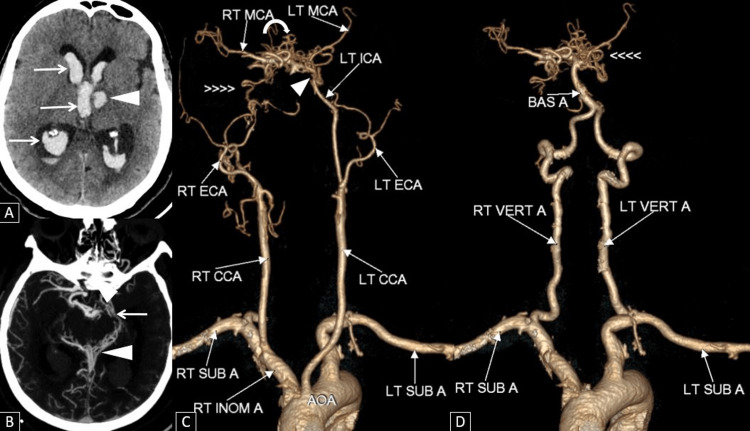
(A) Axial CT brain image showing an acute intraparenchymal hemorrhage in the anterior aspect of the left thalamus (arrowhead) with extensive intraventricular hemorrhage extensions (arrows). (B) Axial reformatted CT angiogram of the brain showing numerous small collaterals in the region of the circle of Willis (arrow) and around the midbrain (arrowhead). (C) Post-processed image of CT angiogram of anterior circulation of the brain showing occluded right internal carotid artery (>>>>), occluded supraclinoid segment of the left internal carotid artery (arrowhead), reformed bilateral MCAs by collaterals (curved arrow) from the vertebrobasilar system. (D) Post-processed image of CT angiogram of posterior circulation of the brain showing mild compensatory dilatation of the basilar artery, and bilateral vertebral arteries with numerous small collaterals in the region of the circle of Willis (<<< LT: Left thalamus, RT: Right thalamus, MCA: Middle cerebral artery, BAS A: Basilar artery, VERT  A: Vertebral arteries, ICA: Internal carotid artery,  CCA: Common carotid artery, ECA: External carotid artery, INOM A: Innominate artery, SUB A: Subclavian artery
